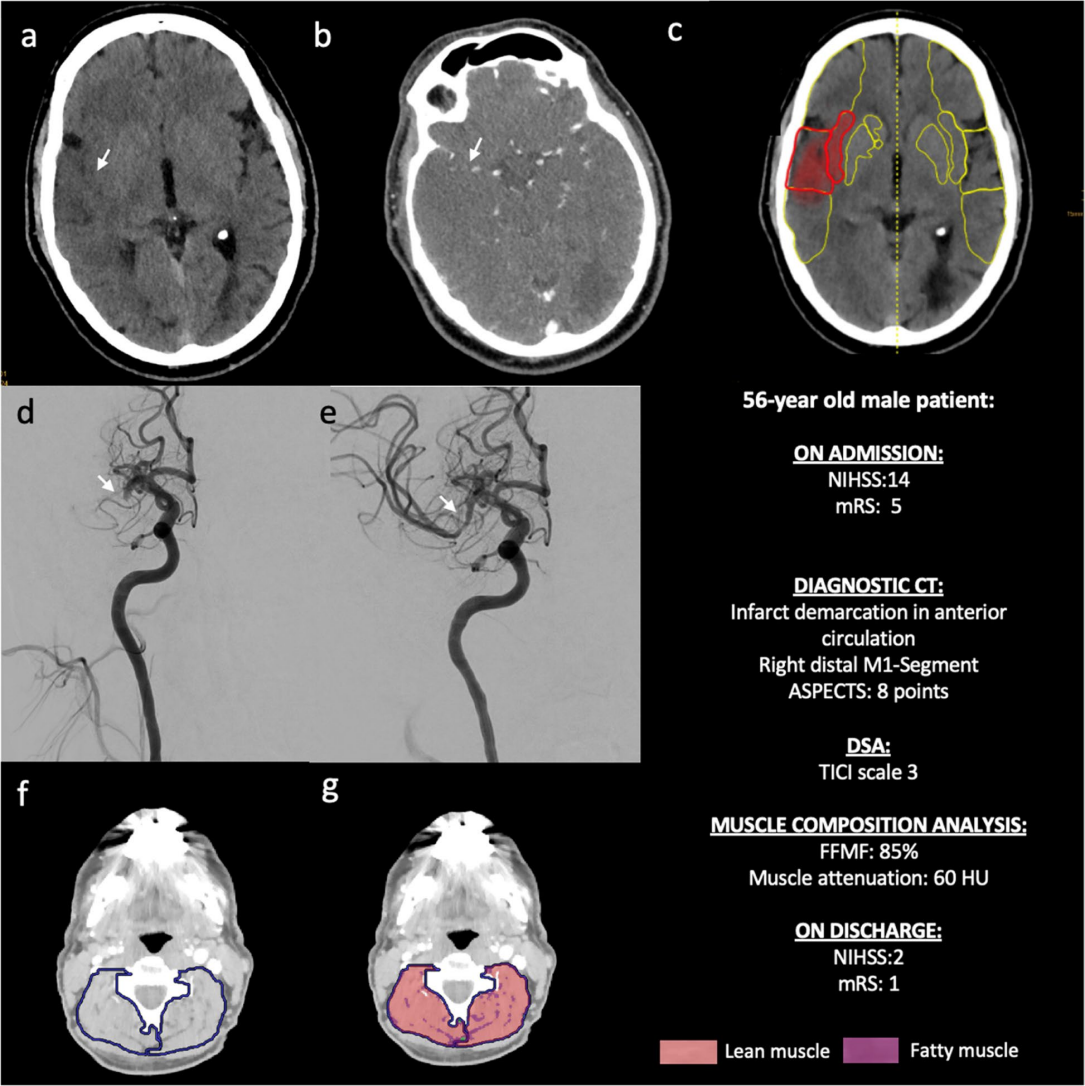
Representative images of a 56-year-old male patient with a high fat-free muscle fraction of 85% and ischemic stroke by right cerebral artery occlusion. Non-enhanced CT scan (a) demonstrates beginning infarct area demarcation (white arrow) in the anterior circulation due to occlusion of the distal M1 segment of the right middle cerebral artery (white arrow, b). Additional analysis of computer-assisted early ischemic changes revealed an ASPECT score of 8 (c). The patient underwent systemic thrombolysis and successful endovascular intervention with mechanical thrombectomy with complete recanalization (TICI scale 3) (white arrows, d and e). Images f and g visualize the assessment of skeletal muscle fat infiltration as previously described
